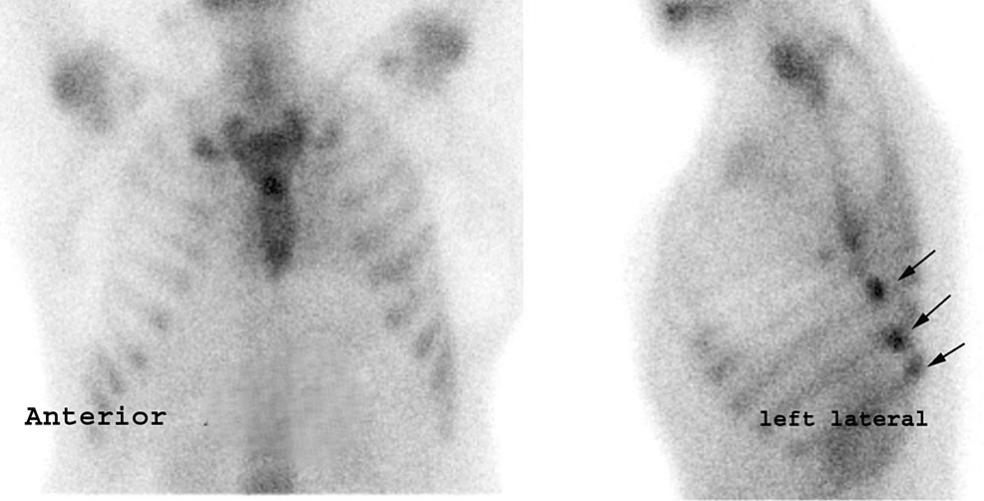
Anterior and left lateral planar Tc-99m PYP scintigraphy Anterior and left lateral planar Tc-99m PYP scintigraphy showing multiple focal rib lesions (arrows), overlapping vertical linear uptake in the left humerus noted superior to the fracture sites. Tc-99m PYP: technetium-99m pyrophosphate
